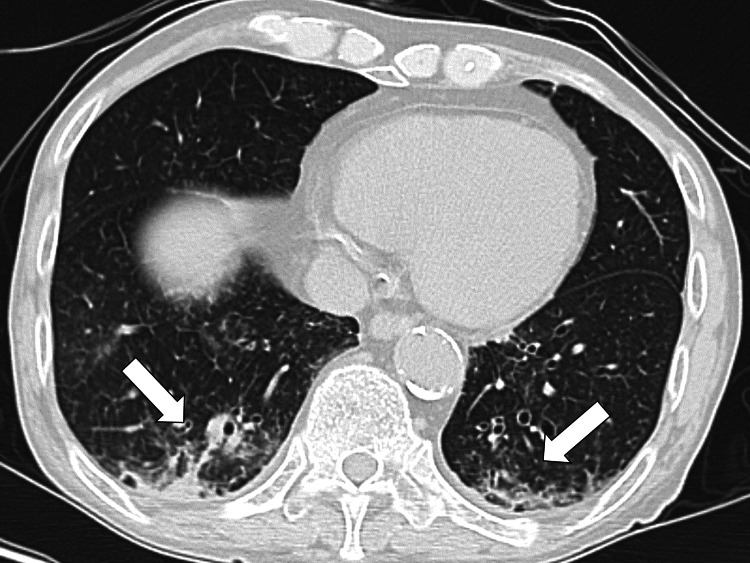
Chest computed tomography showed bilateral interstitial infiltration surrounding the bronchi in the lungs, indicating chronic bronchitis (white arrows).
